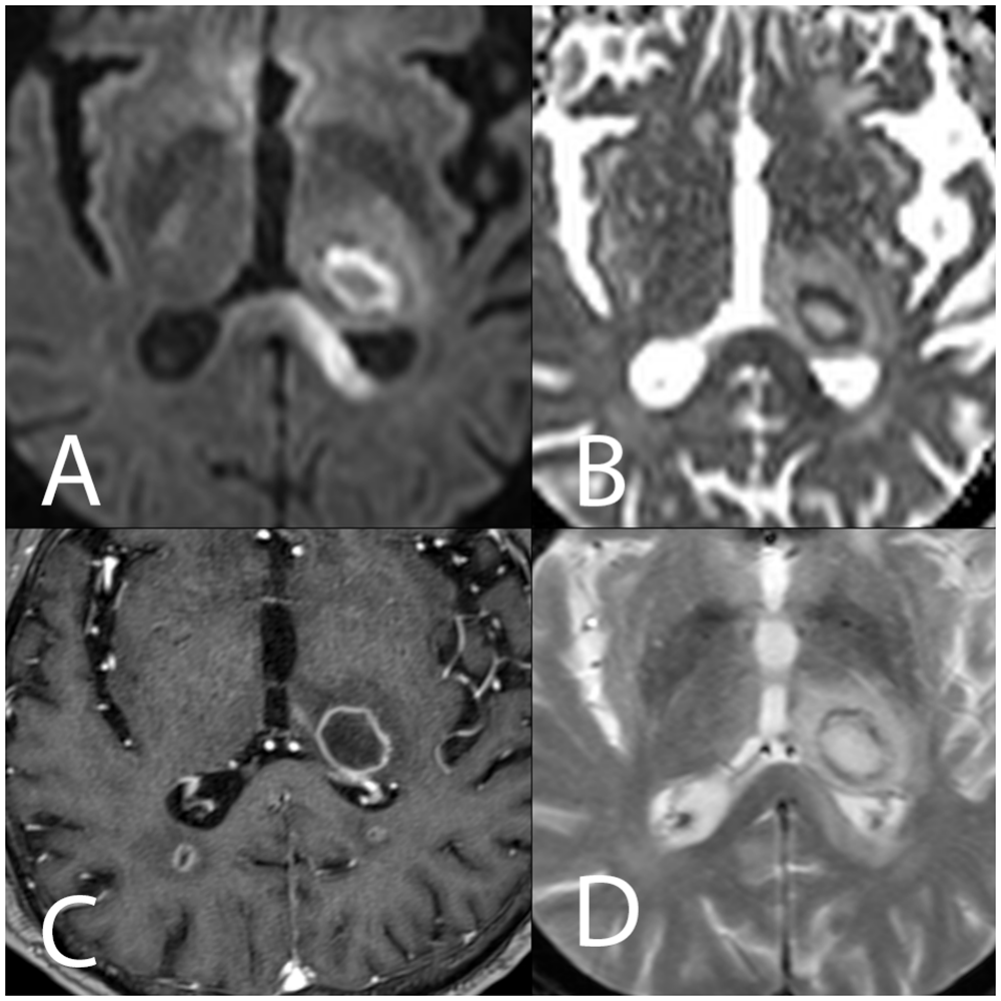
Left thalamic abscess with target-like characteristics (patient #10). The DWI sequence (A) and the ADC cartography (B) show a central hypointense area on DWI (high ADC value), a hyperintense circular area on DWI (low ADC) and a peripheral milder hyperintensity (upper ADC value rim). (C) Annular peripheral enhancement after gadolinium injection on T1W images. (D) Mild hypointense rim on T2*W images.
